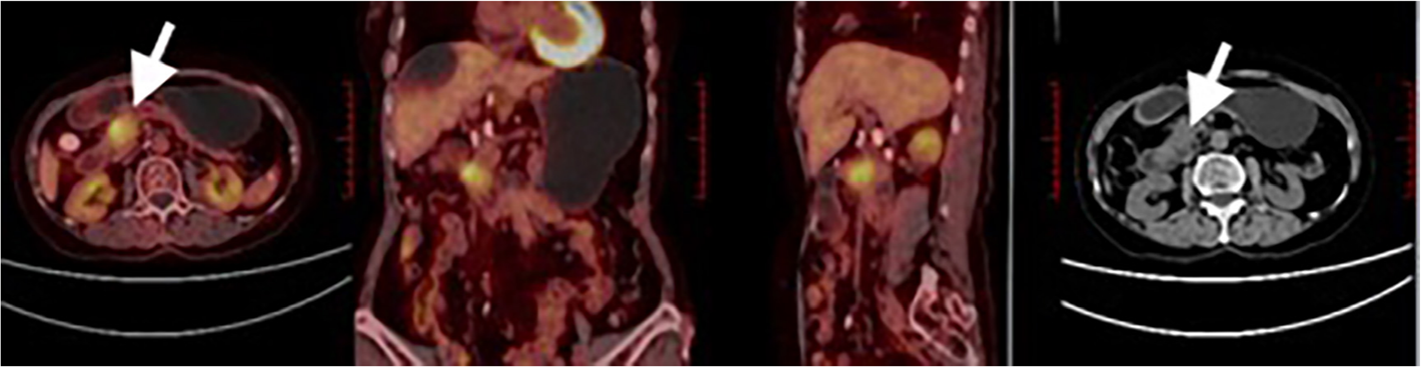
Female, 73 years old, pancreatic head cancer. ^18^F-FDG PET/CT fusion images showed a mass in the pancreatic head, with increased radioactivity uptake. SUV_max_ 6.3, SUV_mean_ 3.6, MTV 5.4 cm^3^, TLG 19.5 g, which were all below the cutoff values. The patient showed good treatment effect with SBRT, alive till the end of follow-up, whose OS and PFS time were 20.3 months and 17.6 months, respectively
